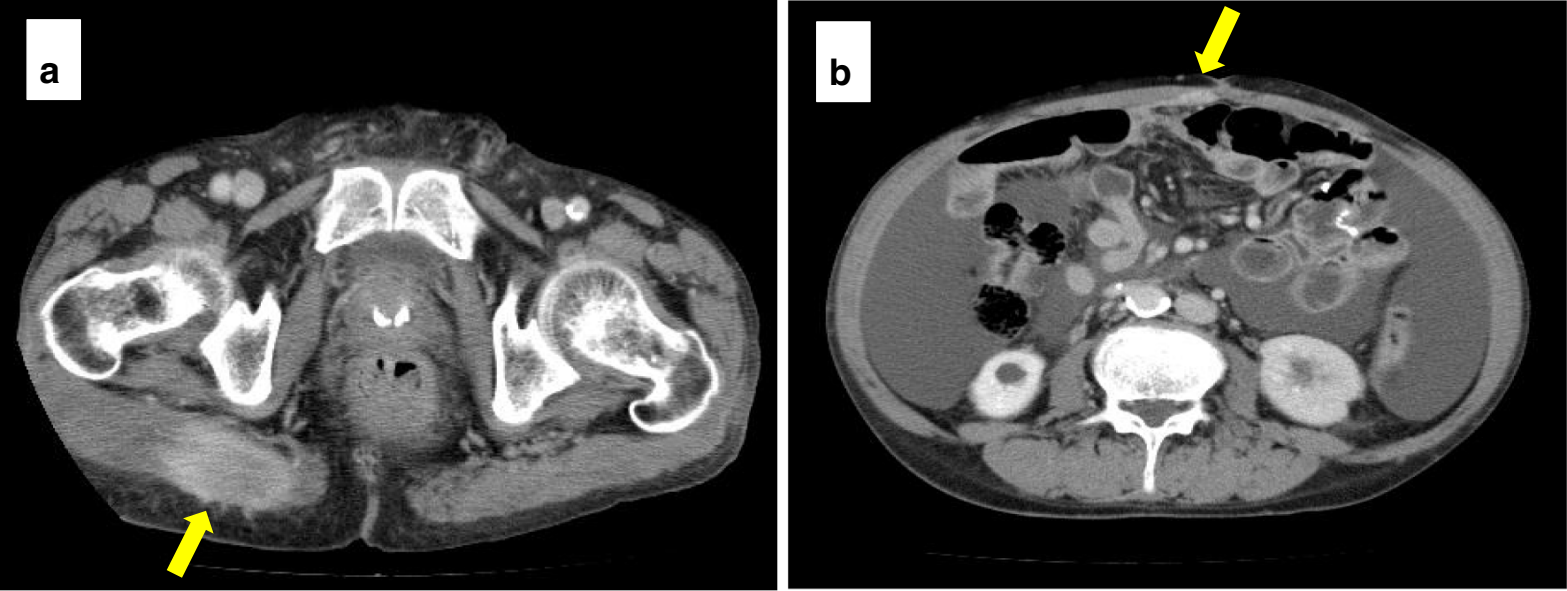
Abdominal contrast-enhanced computed tomography images 6 months after gastrectomy. a Computed tomography scan shows a solid mass (arrows) measuring 52 mm × 28 mm in the right gluteal muscle. b Computed tomography scan shows a disseminated nodule on the abdominal wall (arrows) and massive ascites
